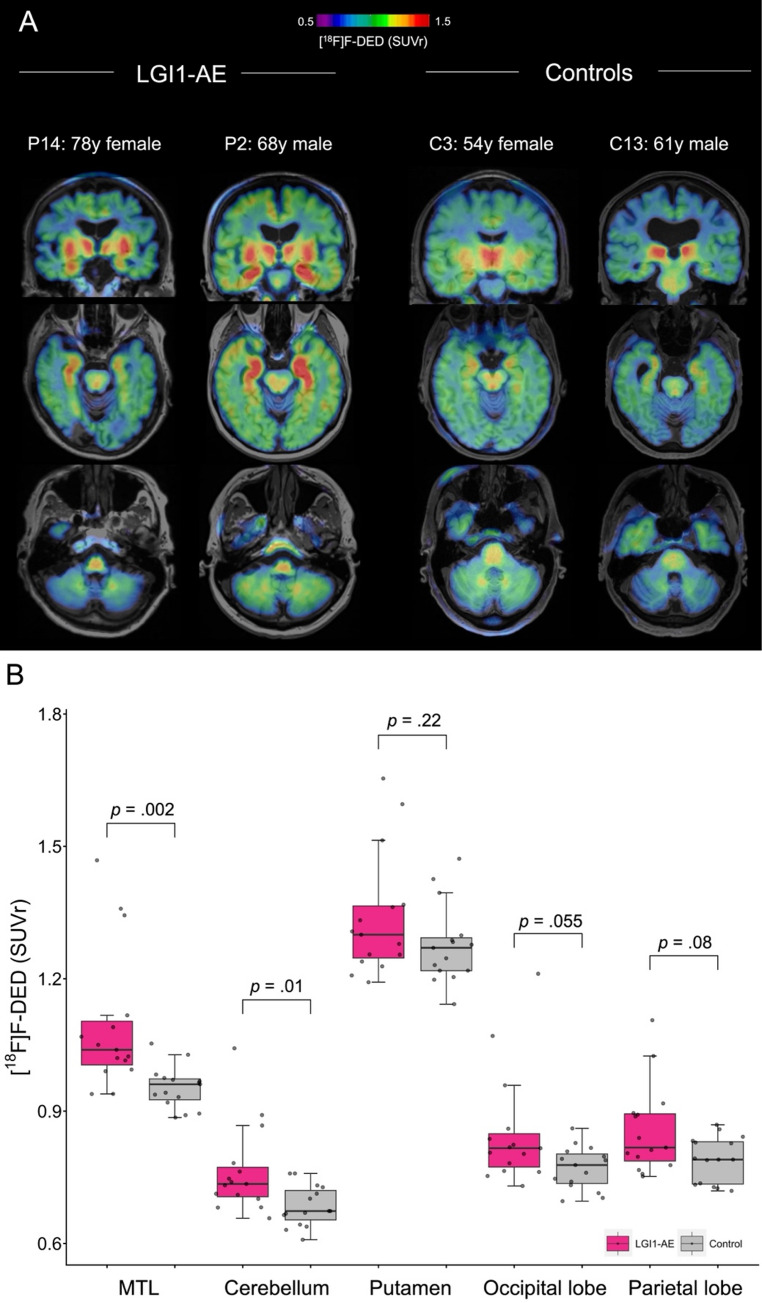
[^18^ F]F-DED PET detects astrogliosis in LGl1-AE patients (A) Coronal and axial planes of [^18^F]F-DED PET images (SUVr, referenced to straight gyrus) fused with individual MRI (T1 weighted) show two examples of LGI1-AE patients (mild and severe PET signal alteration) and two controls. All 15 LGI1-AE patients and 15 controls are displayed in the Supplement. (B) Quantitative [^18^F]F-DED PET SUVr (referenced to straight gyrus) in comparison of 15 LGI1-AE patients and 15 controls in different regions of interest: medial temporal lobe (MTL), cerebellum, putamen, occipital lobe and parietal lobe. Wilcoxon rank-sum test. FDR correction for *n* = 5 target regions
